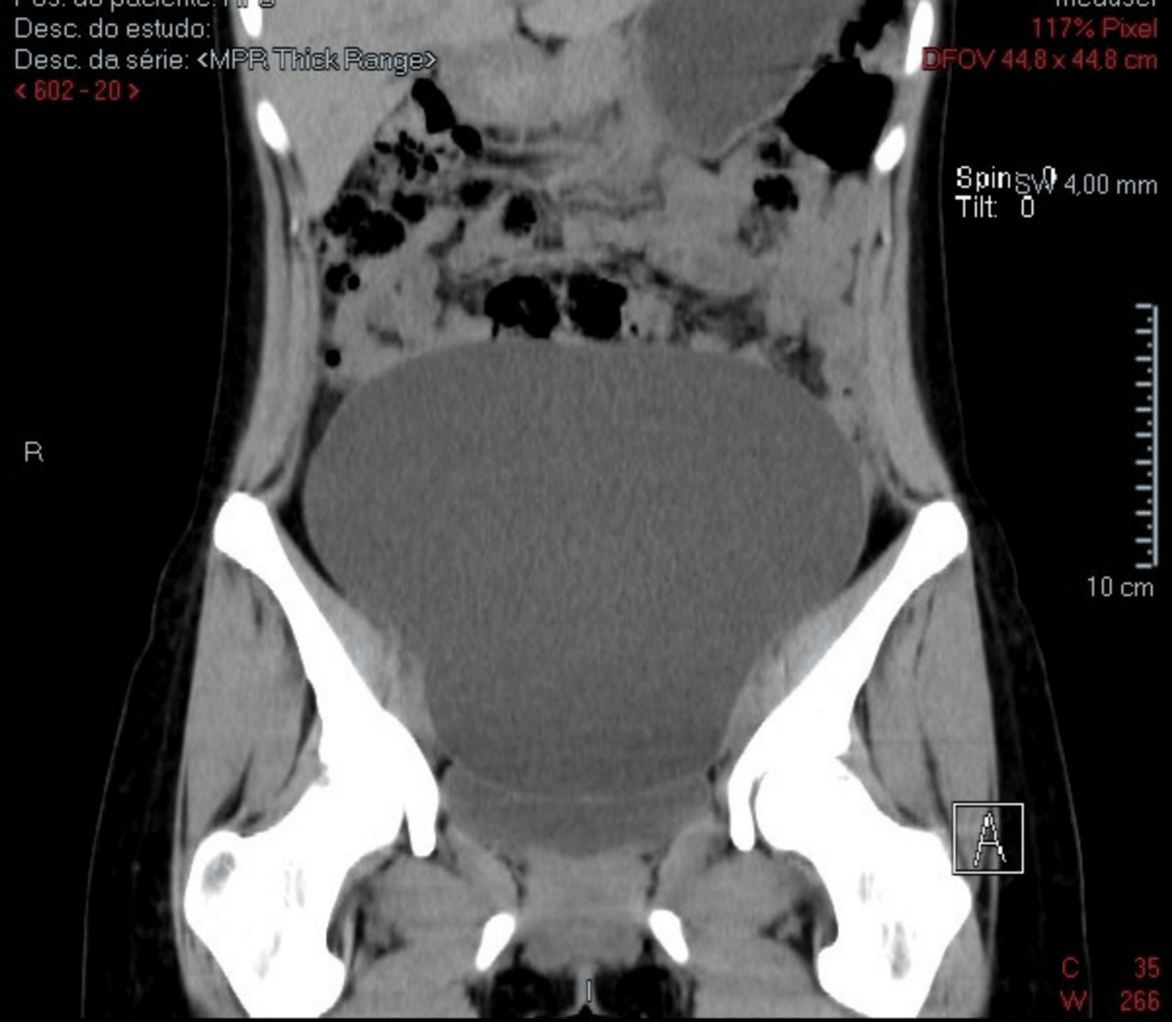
Abdominopelvic CT scan (coronal section) This image demonstrates a clear separation between the bladder and the cyst. CT: computed tomography
